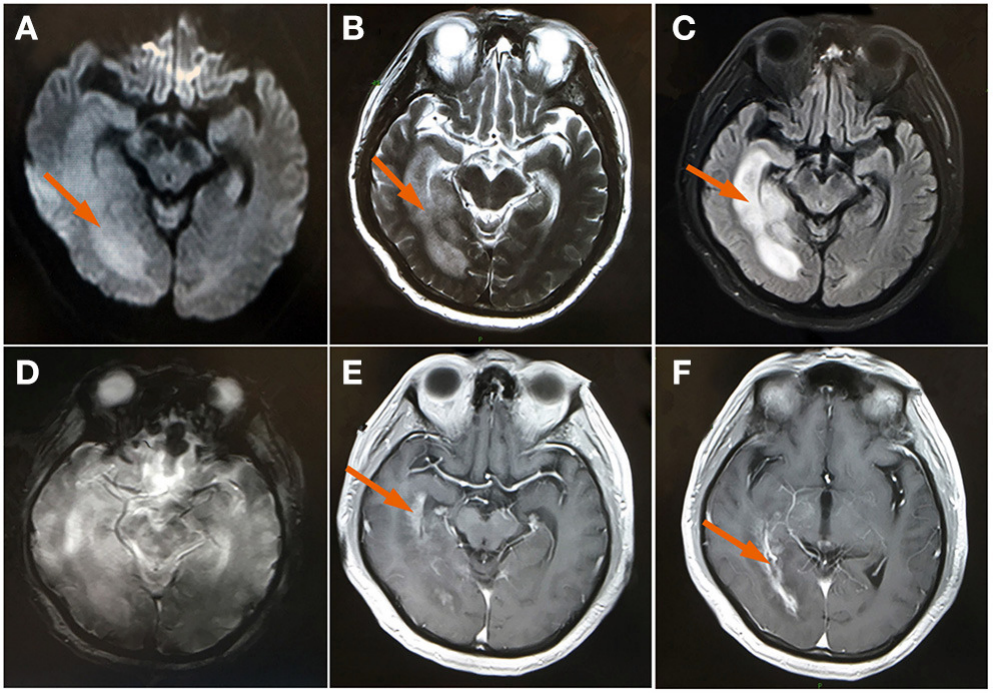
MRI brain (A) Axial DWI and (B) axial T2WI showed a high signal and the size of the edematous area showed a marked increase at the right temporal occipital lobe (arrow); (C) FLAIR image showed a swollen, edematous temporal occipital lobe (arrow); (D) axial GRE sequence at the same level with the high signal of T2WI; (E,F) A contrast-enhanced image demonstrated an abnormal enhancement of the right temporal occipital lobe and bilateral choroid (arrow). DWI, diffusion-weighted imaging; FLAIR, fluid-attenuated inversion-recovery; GRE, gradient echo image; T2WI, T2 weighted image.
